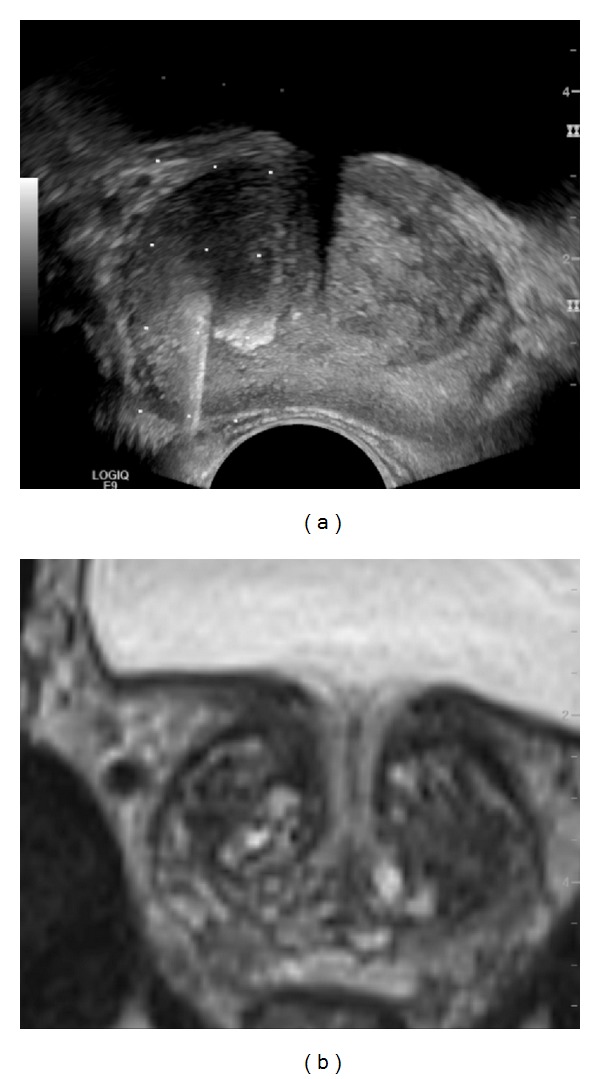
Ultrasound/mpMRI image fusion guided biopsy of the right peripheral zone of the prostate: low signal intensity nodule on T2-weighted image (b) directly biopsied under live-ultrasound guidance (a).
